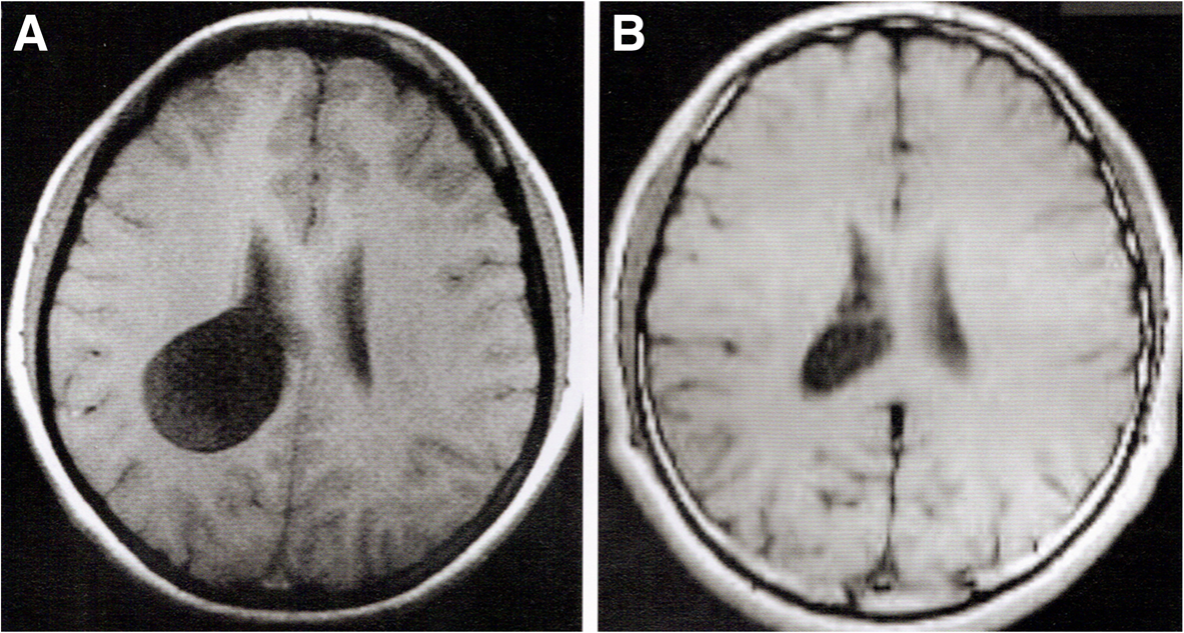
MR image of a cyst was diagnosed in the lateral ventricle (A) before operation and (B) follow-up 3-months after operation.
